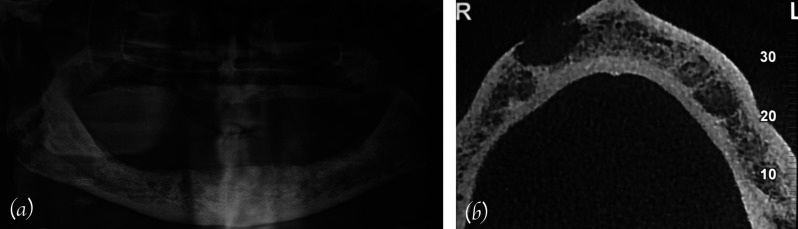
a) OPT showing no specific signs of bone alterations; b) CT scan showing mandibular destruction around the mental foramen.
